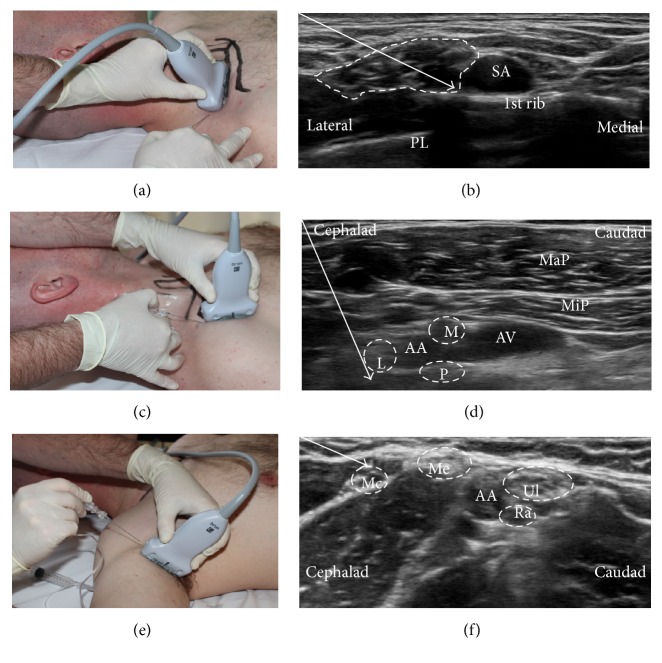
Ultrasound-guided brachial plexus blocks using a short axis, in-plane technique. Supraclavicular brachial plexus block (a) with the transducer parallel with the clavicle and with needle tip positioned subfascial and intracluster injection (b). Infraclavicular brachial plexus block (c) with the transducer in the deltopectoral groove and with needle tip position (d) close to the axillary artery (AA) at each of the cords, that is, lateral (L), medial (M), and posterior (P) cord. Axillary brachial plexus block (e) with needle tip position (f) at each of the four individual nerves, that is, musculocutaneous (Mc), median (Me), ulnar (Ul), and radial (Ra) nerve. Pl: pleura; MaP: major pectoral muscle; MiP: minor pectoral muscle; AV: axillary vein.
